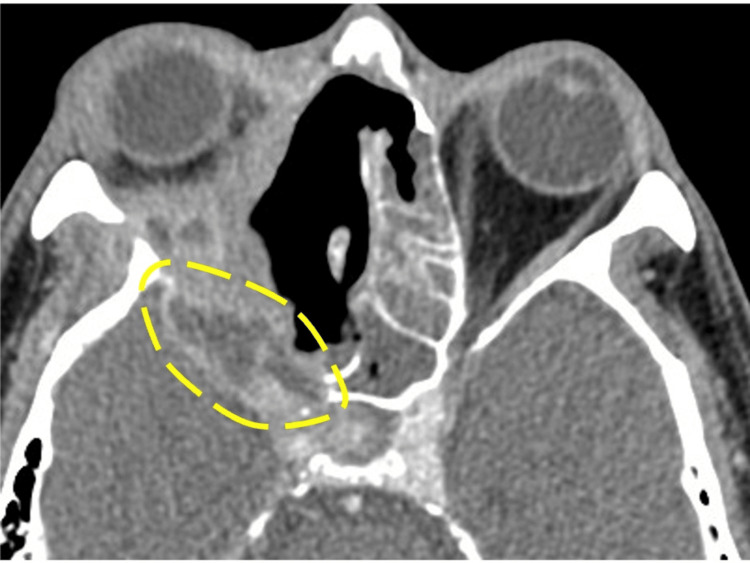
Contrast-enhanced CT image of the sinus immediately after surgery (axial section) The lesion at the tip of the orbit remained (dotted line).
